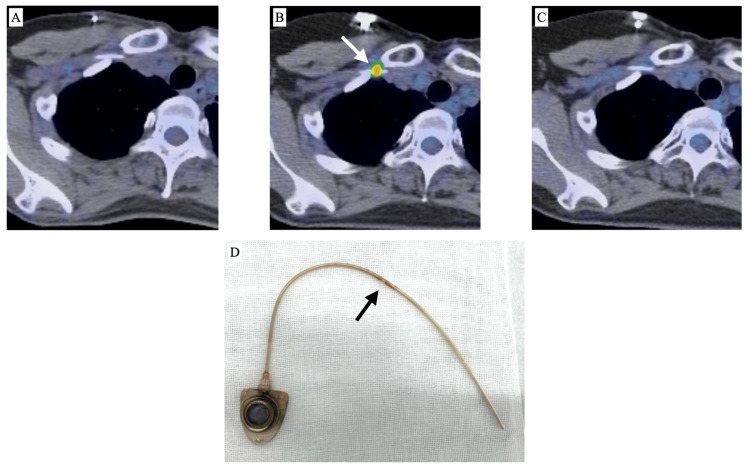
FDG-PET/CT scans and TIVAD of a 40-year-old woman with malignant lymphoma A:  FDG-PET/CT 159 days after TIVAD implantation shows no abnormal accumulation; B: Increased accumulation observed at the catheter penetration site into the subclavian vein (arrow) 560 days after TIVAD implantation; C: Increased accumulation disappeared on FDG-PET/CT 929 days after TIVAD implantation; D: The TIVAD was removed at the end of chemotherapy. A small amount of thrombus-like deposit is observed corresponding to the catheter penetration site (arrow). FDG: Fluorodeoxyglucose, TIVAD: Totally implantable venous access device
